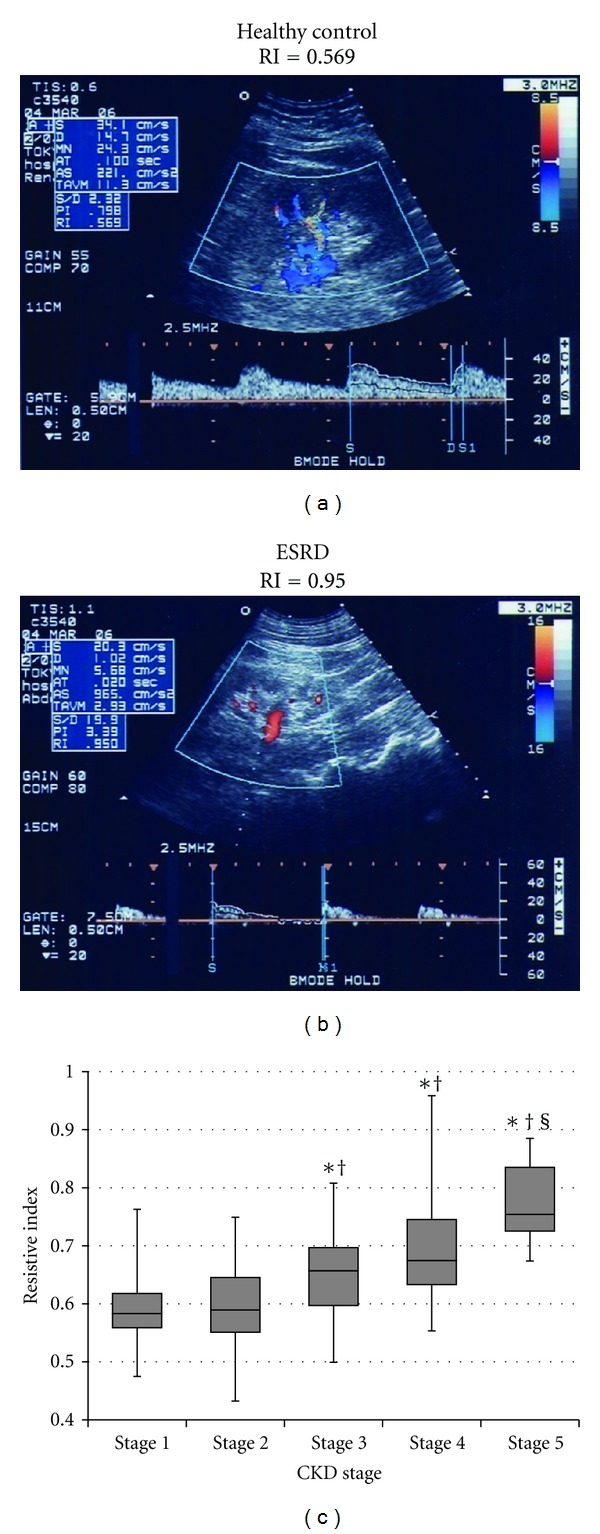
The resistive index (RI) based on CKD stages. An ultrasonographic image of a healthy control with CKD stage 1 (a) and of a patient with end-stage renal disease (ESRD) with CKD stage 5 (b). RI increased with CKD progression (*P* < 0.001) (c). P* < 0.01 versus stage 1, ^†^
